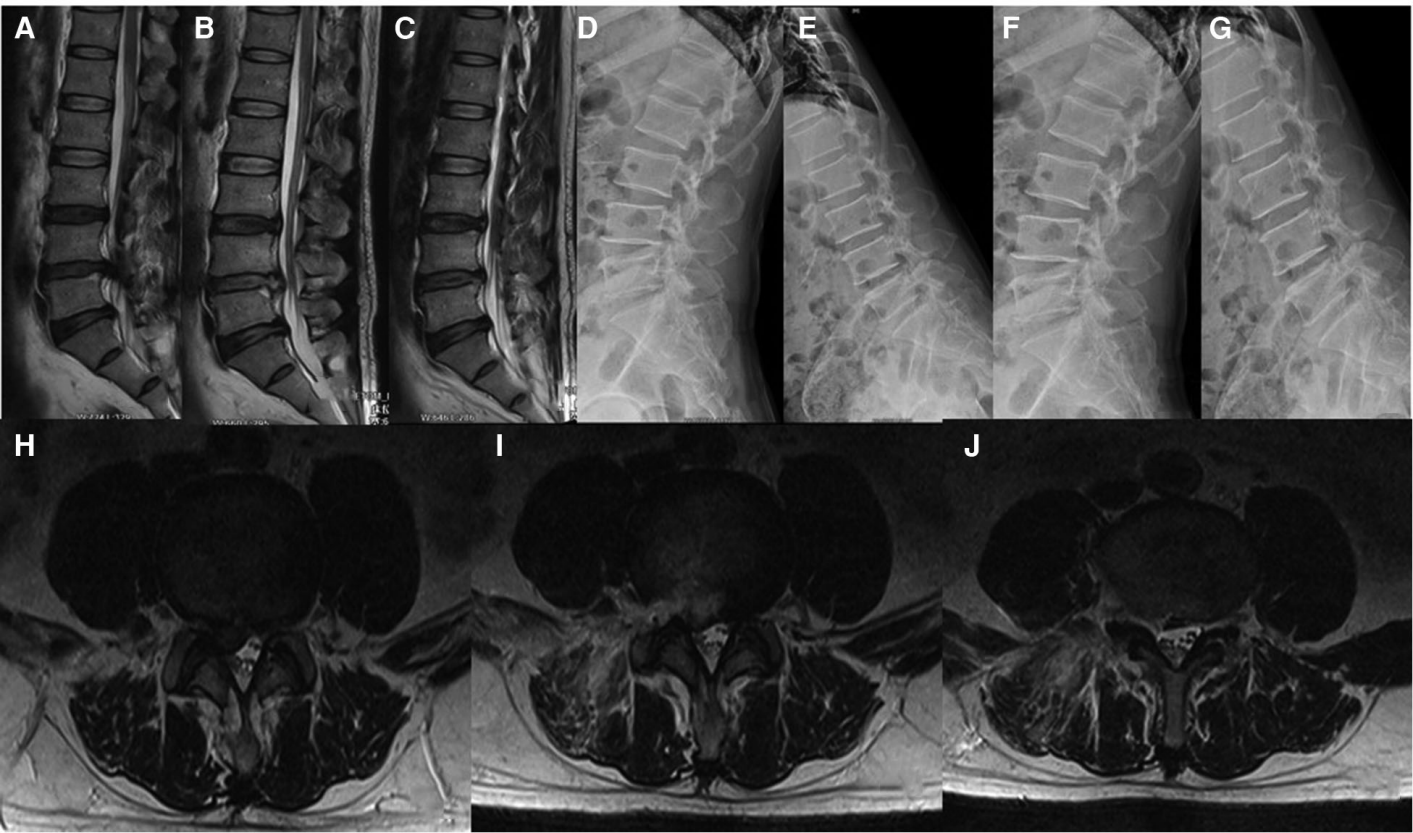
(A) preoperative MRI in sagittal position; (B) sagittal MRI 2 days after surgery; (C) sagittal MRI 6.5 years after surgery; (D,E) preoperative x-ray radiograph in lumbar dynamic position showing no lumbar instability or spondylolysis; (F,G) x-ray radiograph in lumbar dynamic position 6.5 years after surgery showing no lumbar instability;(H) preoperative MRI in coronal position; (I) coronal MRI 2 days after surgery; (J) coronal MRI 6.5 years after surgery.
